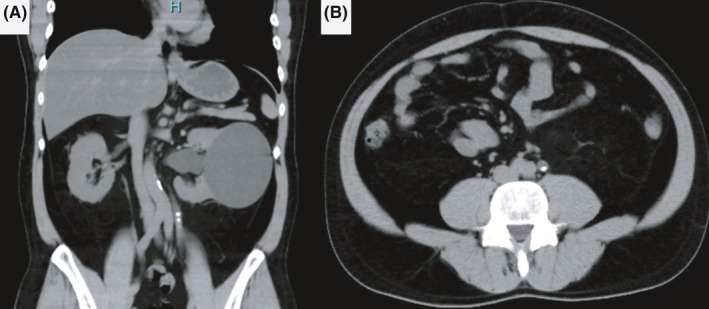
A, Coronal and (B) axial CT images after initial lithotripsy
